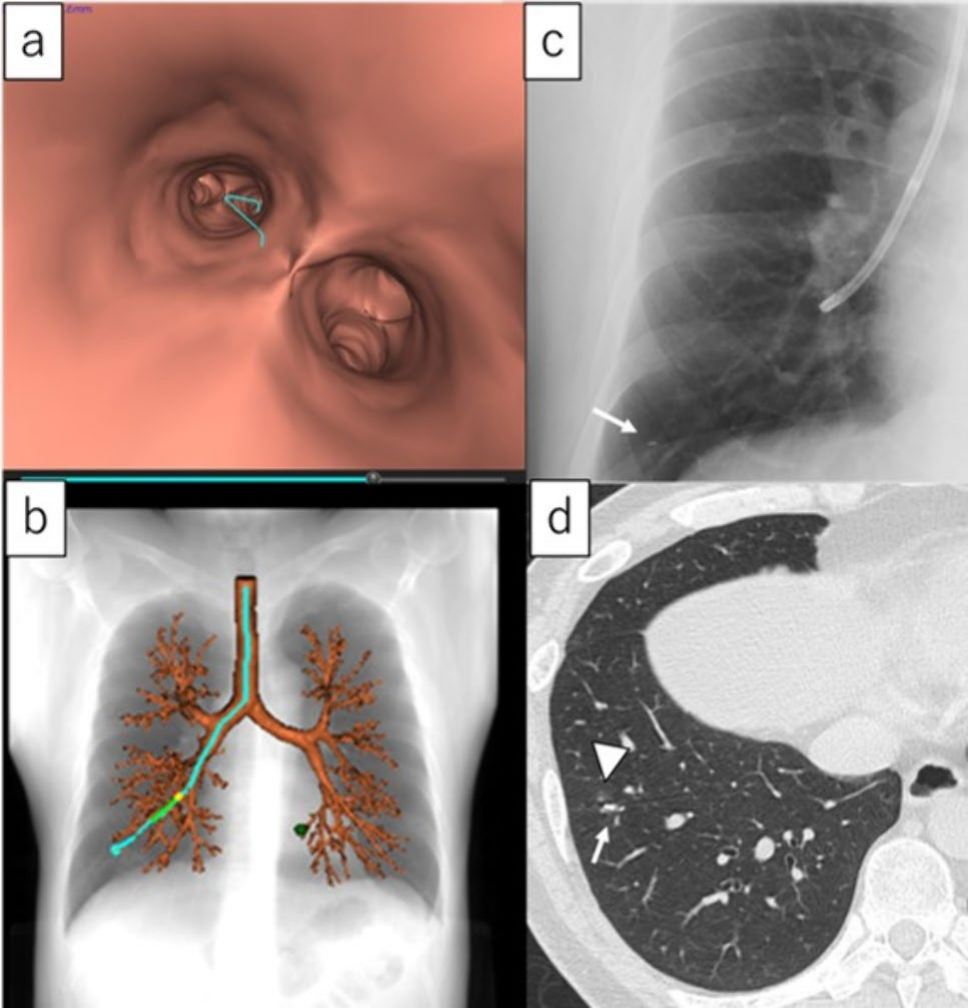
Virtual bronchoscopic image and bronchoscopy for RFID tag placement. The same patients presented in Fig. 2. a, b A virtual bronchoscopic pathway for the target site is created using a VBN system. c An RFID tag (arrow) is placed at the target site using a thin bronchoscope with fluoroscopy in combination with VBN guidance. d Chest CT image after bronchoscopy reveals the relationship between the actual RFID tag (arrow) location and target lesion. The actual RFID tag was located on the dorsal side of the tumour (arrowhead), which was judged to be ‘complete.’ Three days after RFID tag placement, the patient underwent robot-assisted thoracoscopic surgery. *CT* computed tomography, *RFID* radiofrequency identification, *VBN* virtual bronchoscopic navigation
